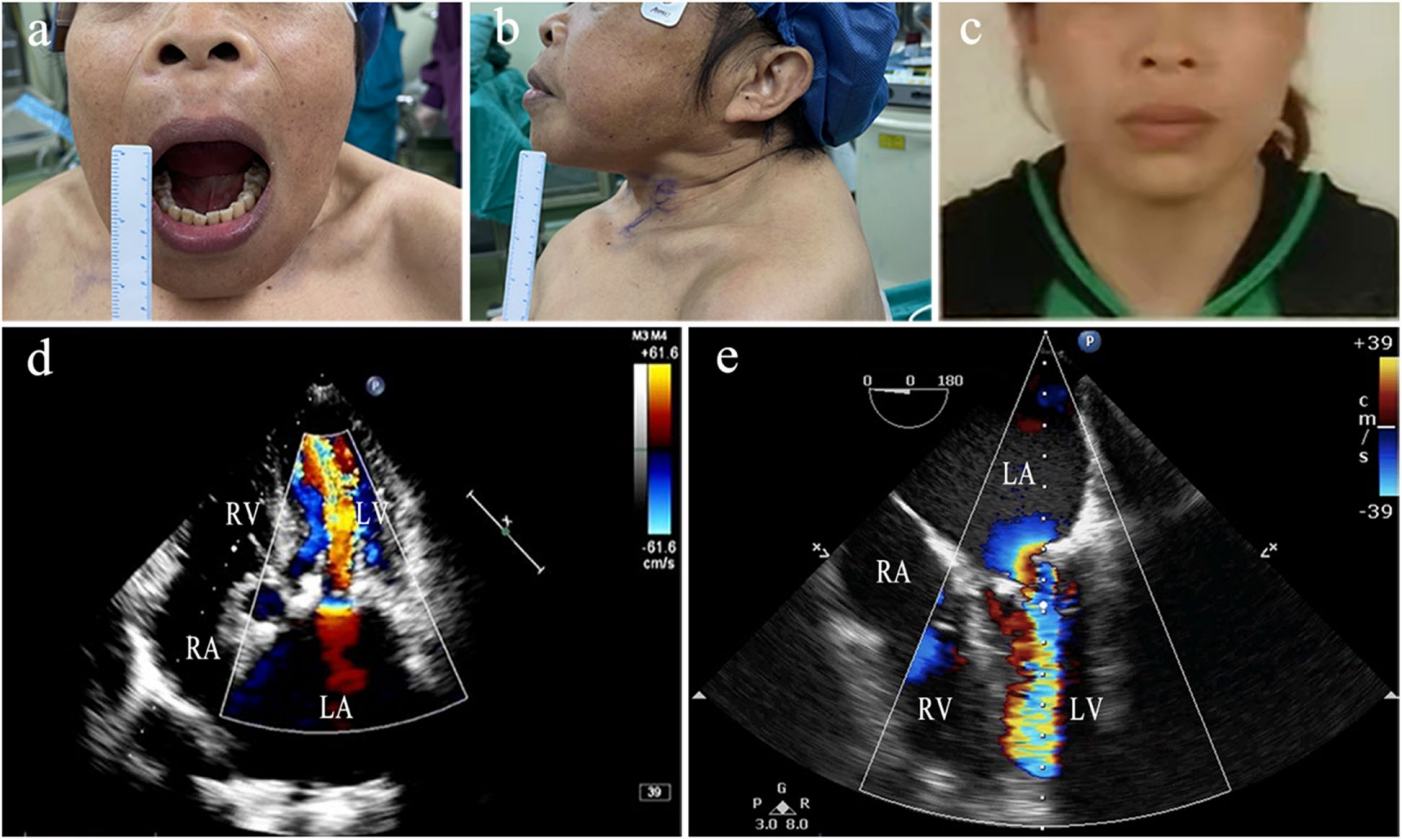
(a, b) Current abnormal human face appearances (deformity of the maxilla and mandible, flattening of nasal cartilage); (c) Previously taken normal facial photograph; (d) Transthoracic echocardiography findings before operation; (e) Transoesophageal echocardiography findings after induction of anaesthesia
